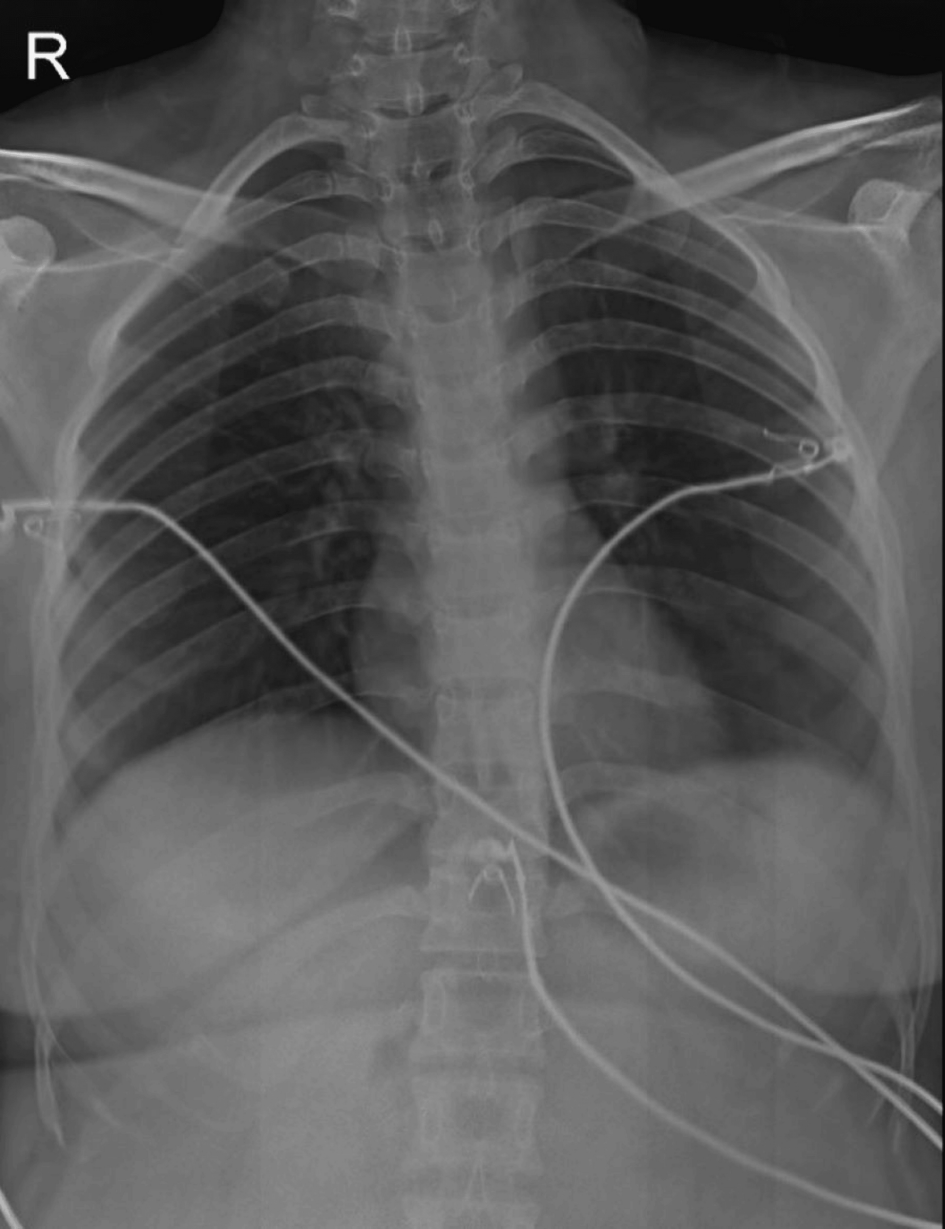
Chest radiograph of the patient on presentation
